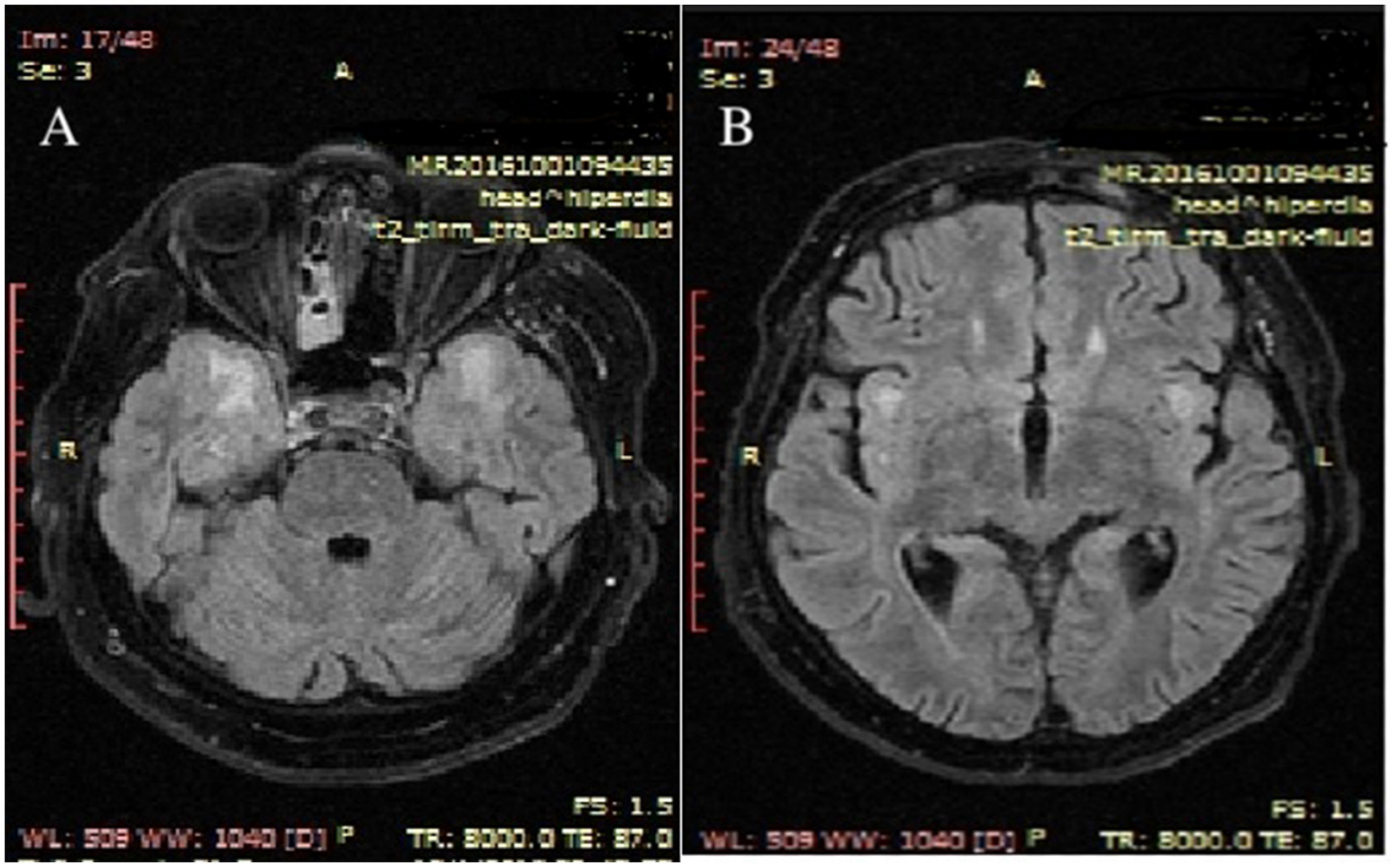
Upon admission to the hospital, MRI brain scans with contrast revealed T2 axial hypersignal in the subcortical white matter of both temporal lobes (A) and in the bilateral insula of the Reil lobes (B). MRI stands for magnetic resonance imaging.
